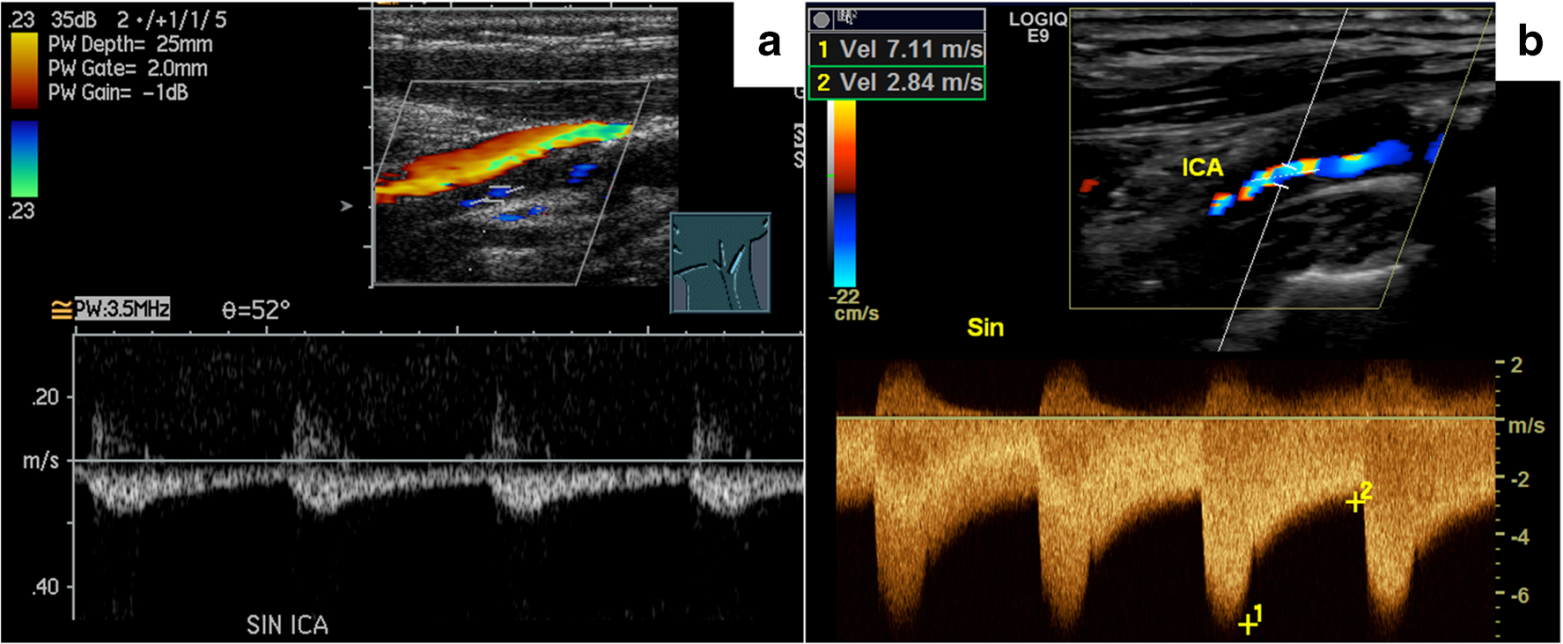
Ultrasound findings in two cases with near-occlusion. a Severe stenosis with low stenosis velocity, distinguishable from conventional stenosis by the low velocity. b Severe stenosis with high stenosis velocity, difficult to distinguish from conventional stenosis
